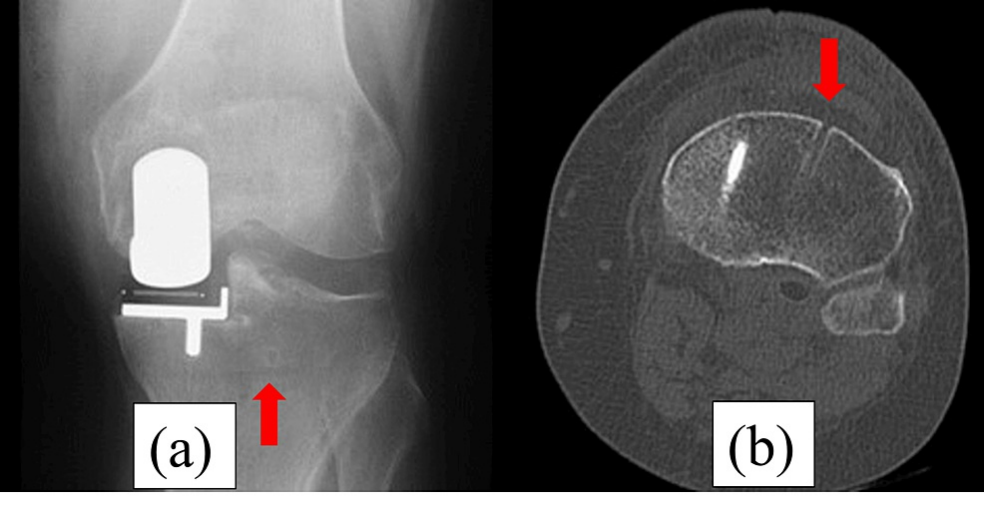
Immediately postoperative radiography and CT. (a) Postoperative radiography shows no fracture line. (b) Postoperative CT shows the level of a pinhole. The red arrows show the place of the pin. The pin was placed outside the tibial mechanical axis.
